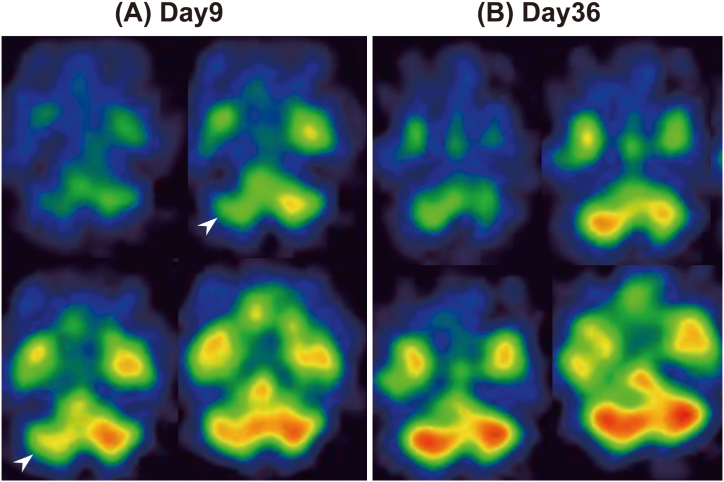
Single photon emission computed tomography using N-Isopropyl-p-[123I] iodoamphetamine (123I-IMP) perfusion scans on days 9 and 36. (A) On day 9, SPECT showed decreased CBF in the right cerebellar hemispheres (arrowhead). (B) On day 36, areas of hypoperfusion had disappeared.
